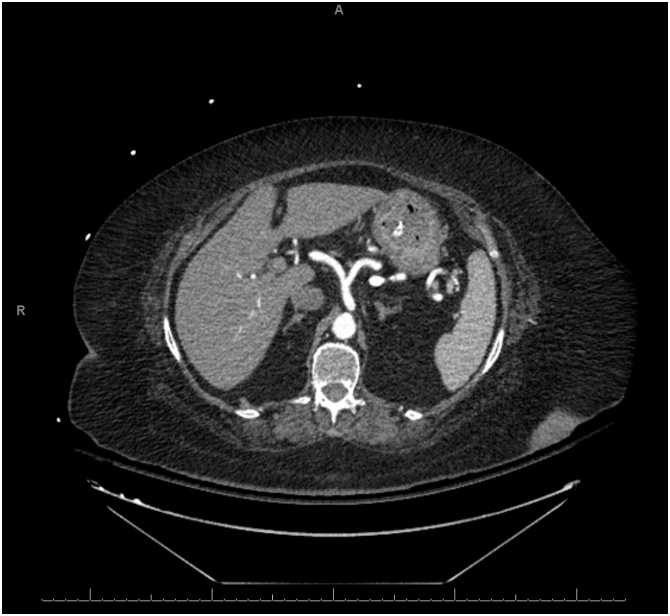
Computed tomography scan of the abdomen showing resolution of the gastric pneumatosis after 4 days.
